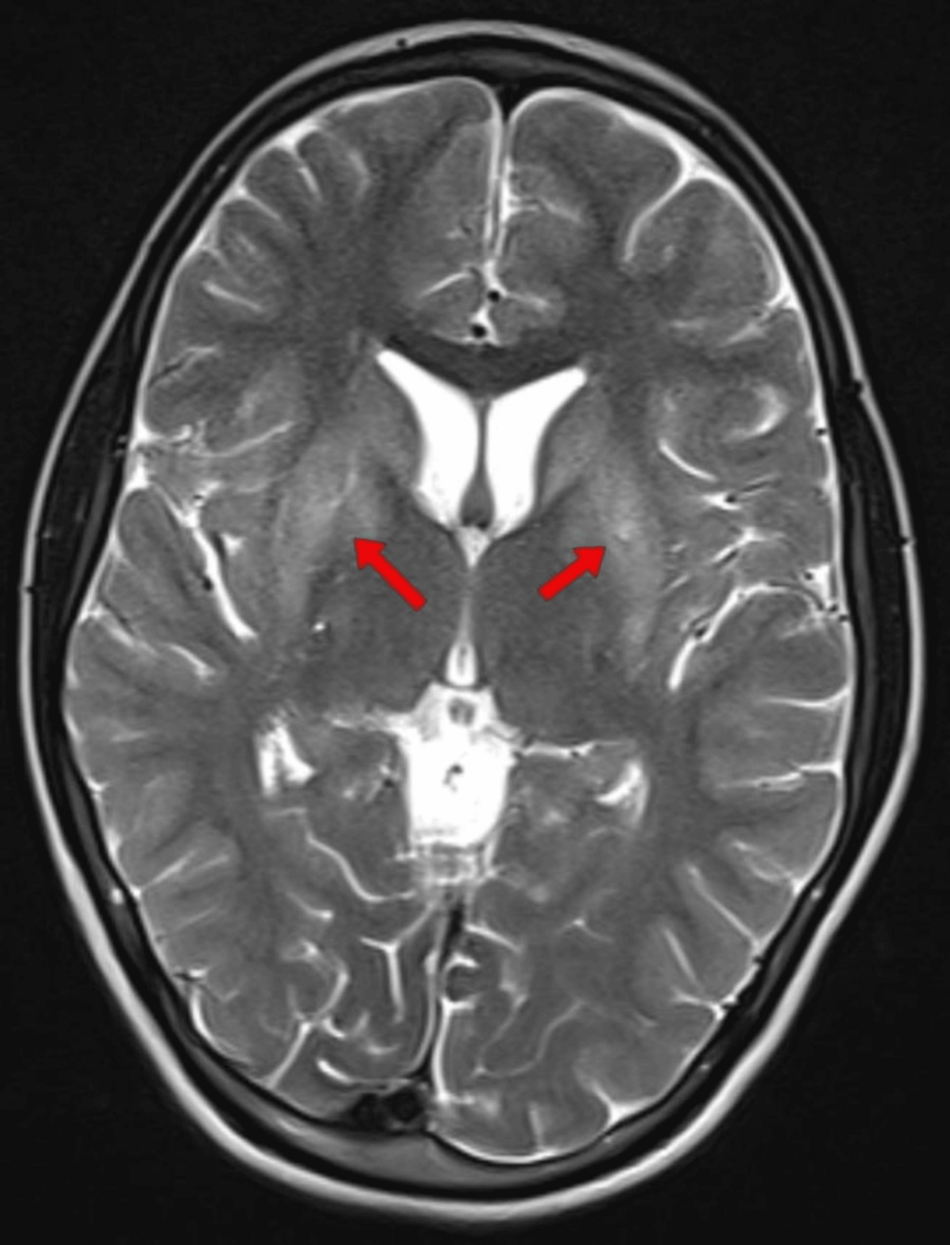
MRI brain of the youngest sibling MRI, Magnetic Resonance Imaging; T2-weighted image of the brain showing bilateral basal ganglia hyperintensity (red arrows).
